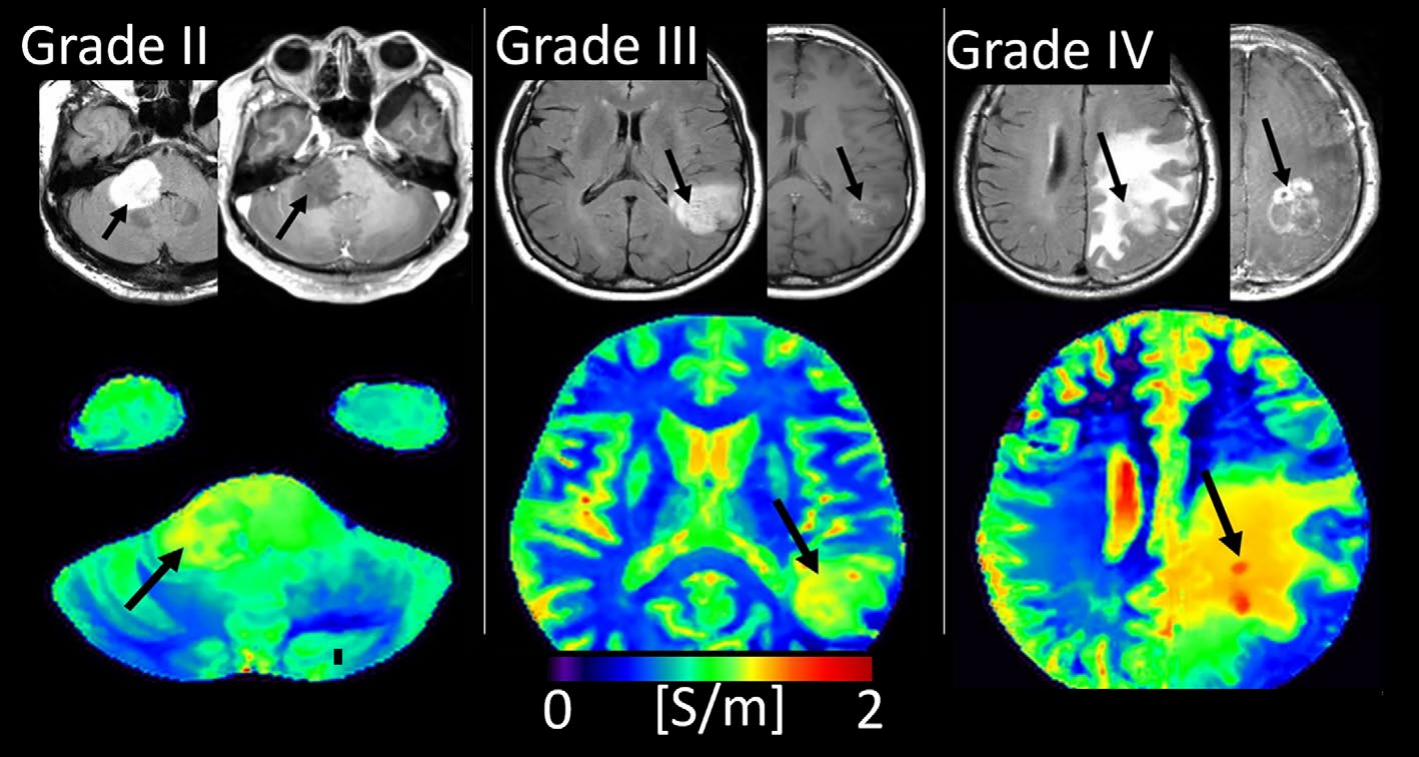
Conductivity maps of diffuse glioma. Grade II (left) to Grade IV (right) according to 2016 WHO classification of Central Nervous System tumors. The upper row shows axial FLAIR (left) and contrast‐enhanced T1‐weighted images (right), and the lower row shows the corresponding conductivity maps. Tumors are indicated by black arrows.
